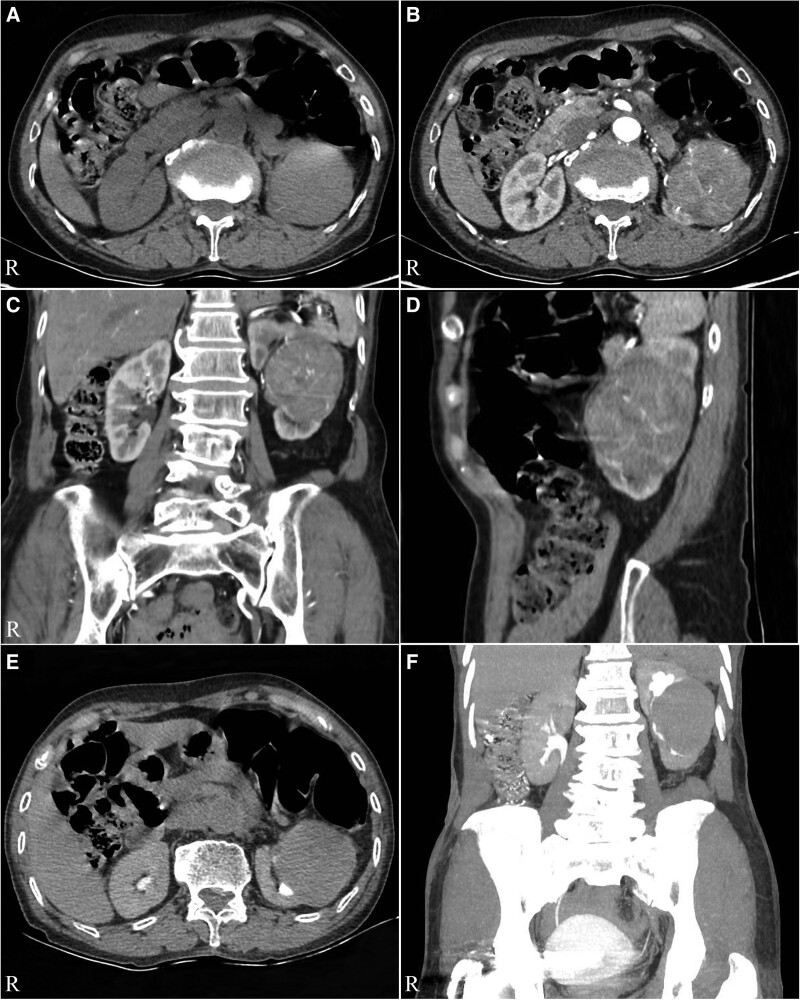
(A) CT indicated a mass in the lower middle part of the left kidney, about 72 mm × 62 mm in size. (B) CT with enhancement showed that the tumor was strengthened. (C) Coronal plane with enhancement. (D) Sagittal plane with enhancement. (E) CTU showed renal pelvis compression and mild hydronephrosis in the upper calyx. (F) Coronal plane of CTU. CT = computed tomography, CTU = computed tomography urography
